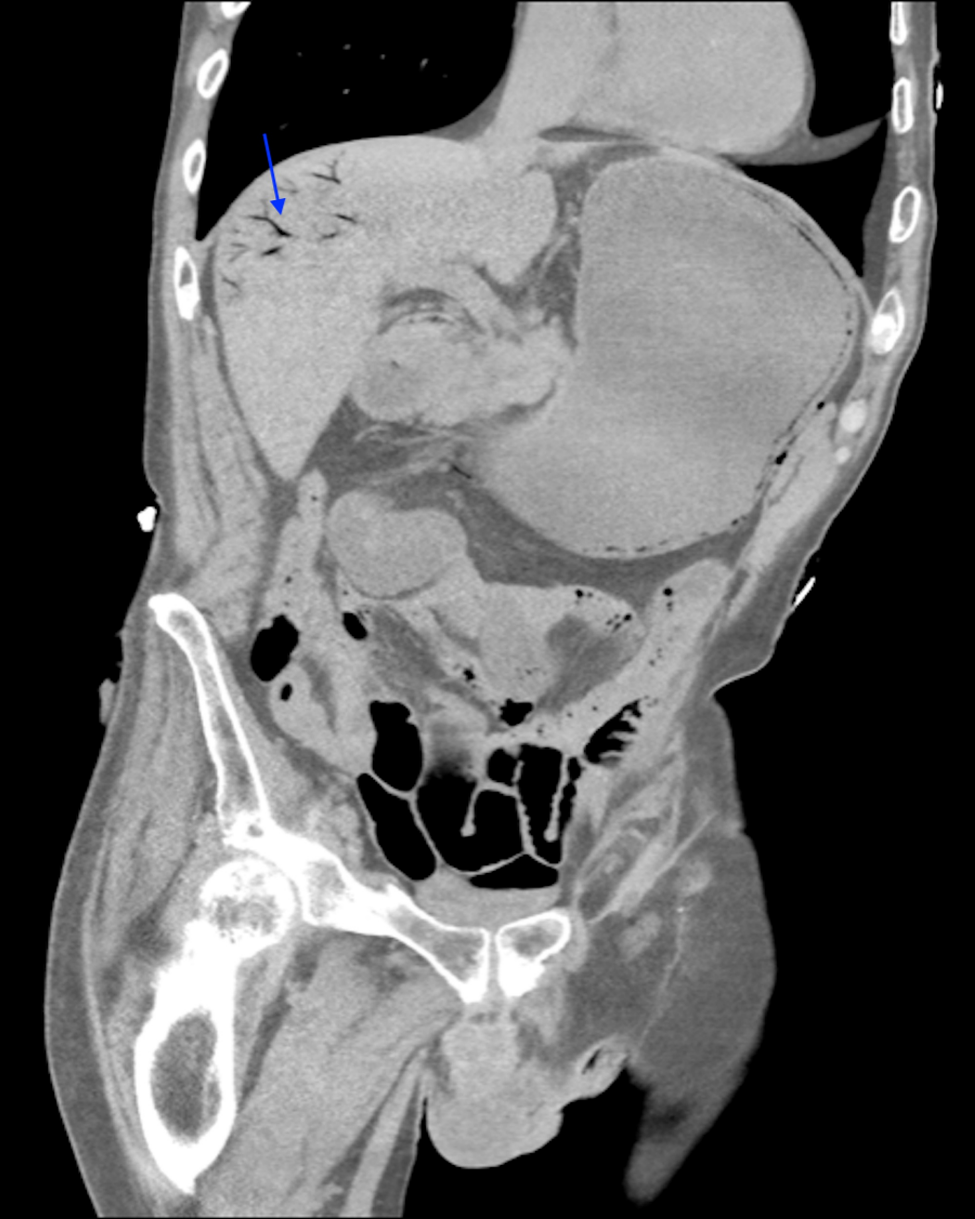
Coronal CT scan of the abdomen and pelvis demonstrating portal gas (blue arrow) and gastric outlet obstruction. CT, computed tomography
